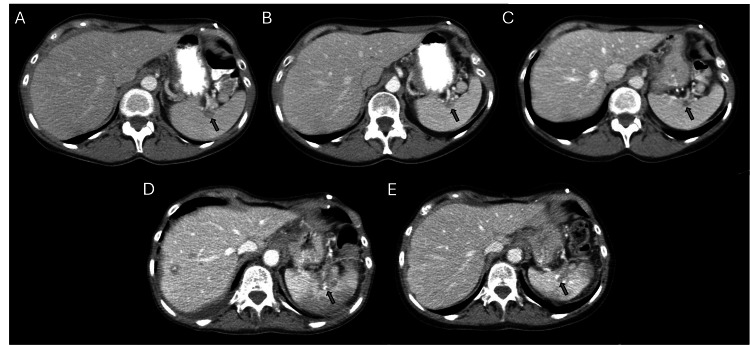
Evolution of the splenic implant during the patient’s disease course. Arrows in each CT A/P image indicate the splenic implant.
